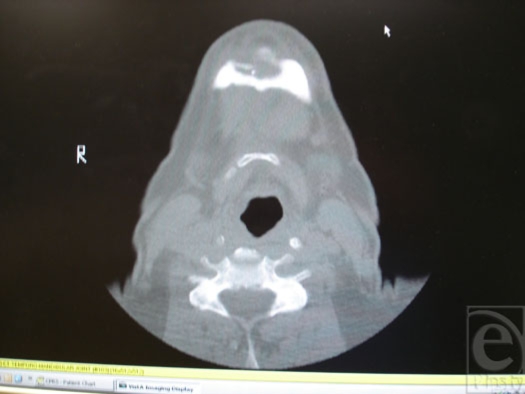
Computed tomographic scan demonstrating infection of mandibular symphyseal bone.
